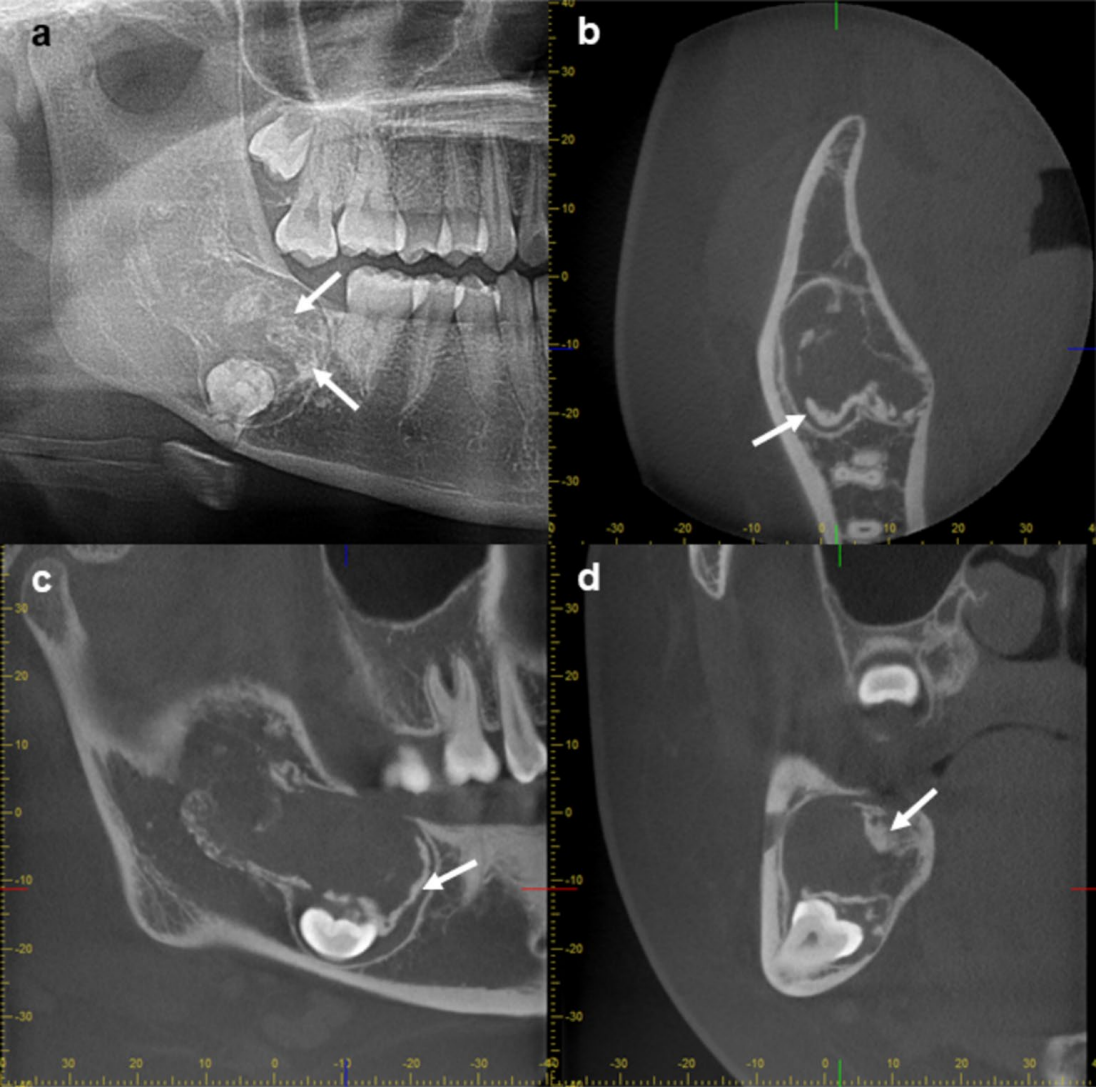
Radiographs of case 6. A preoperative cropped panoramic image (a) and cone beam computed tomography images (b-d) after incisional biopsy show a low attenuation lesion with internal calcification (arrows) associated with an impacted molar tooth
